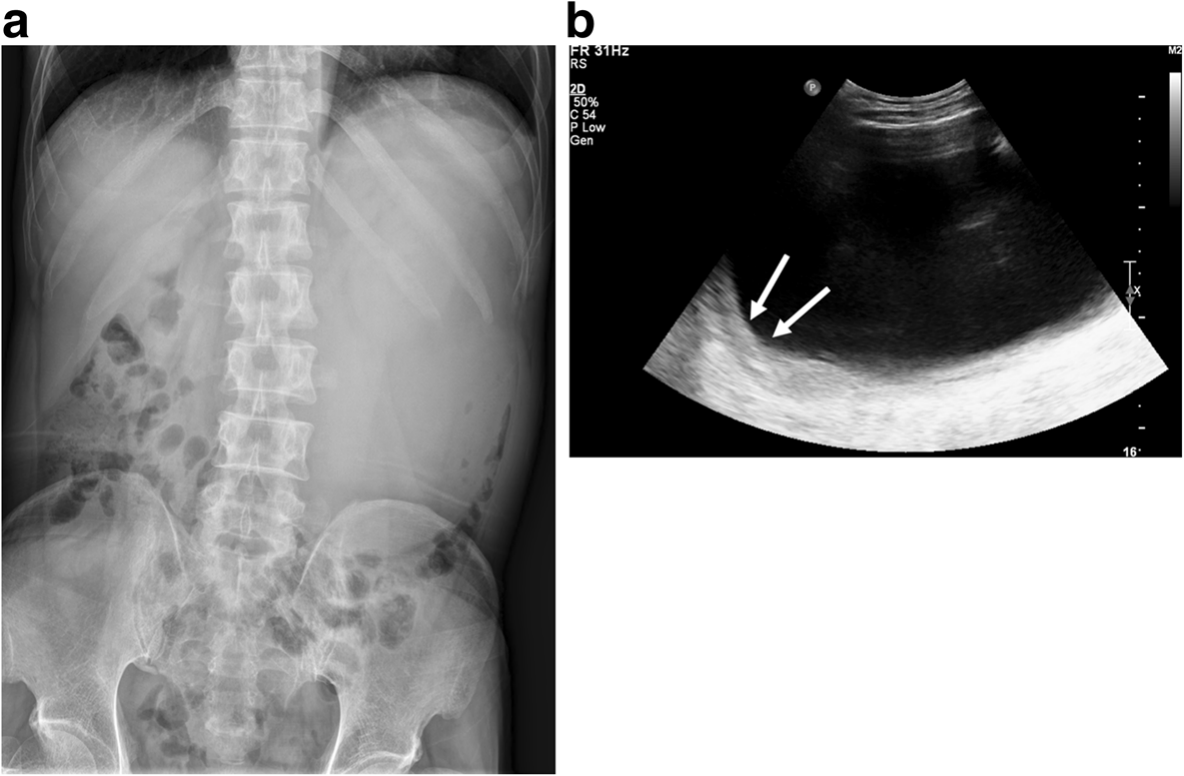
Retroperitoneal desmoid-type fibromatosis in a 46-year-old man. a Abdominal plain radiography shows a large mass-like opacity in the left abdomen. b The ultrasonography shows a large anechoic cystic mass with a thick wall (arrows) without an intracystic solid portion or septum in the left abdomen
